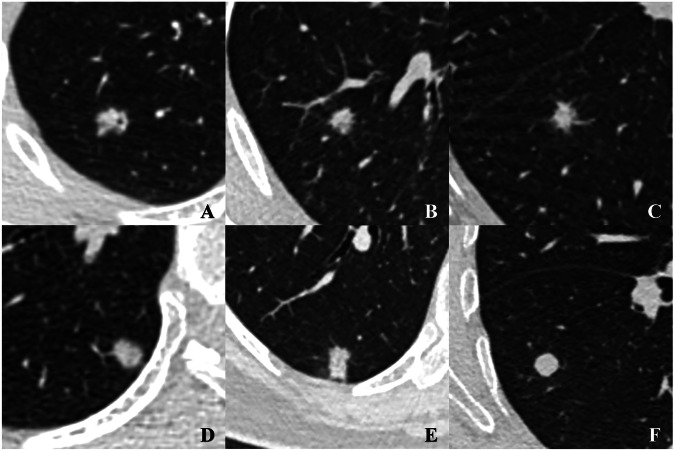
SNs with different density homogeneity. A–E Heterogeneous SNs: these nodules overall exhibit nonuniform soft tissue density on axial CT images, with interspersed regions of relatively high and low attenuation. F Homogeneous SN: this nodule exhibits pure soft tissue attenuation on axial CT image, without any region of relatively low attenuation. SN, solid nodule; CT, computed tomography
